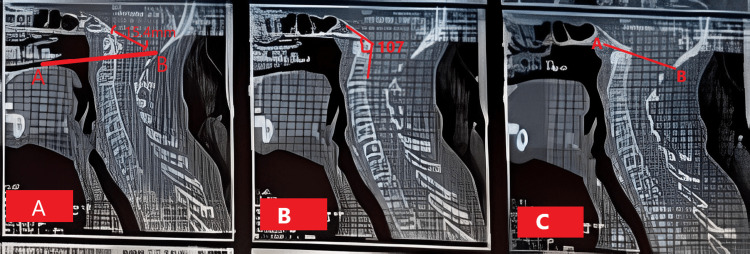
CT image of the cervical spine A: The tip of the odontoid process lies 15.4 mm above Chamberlain’s line; B: The clivus angle measures 107 degrees; C: The tip of the dens tends to transect Wackenheim’s canal and lies 2 mm below Mc Rae’s line. CT: computed tomography
